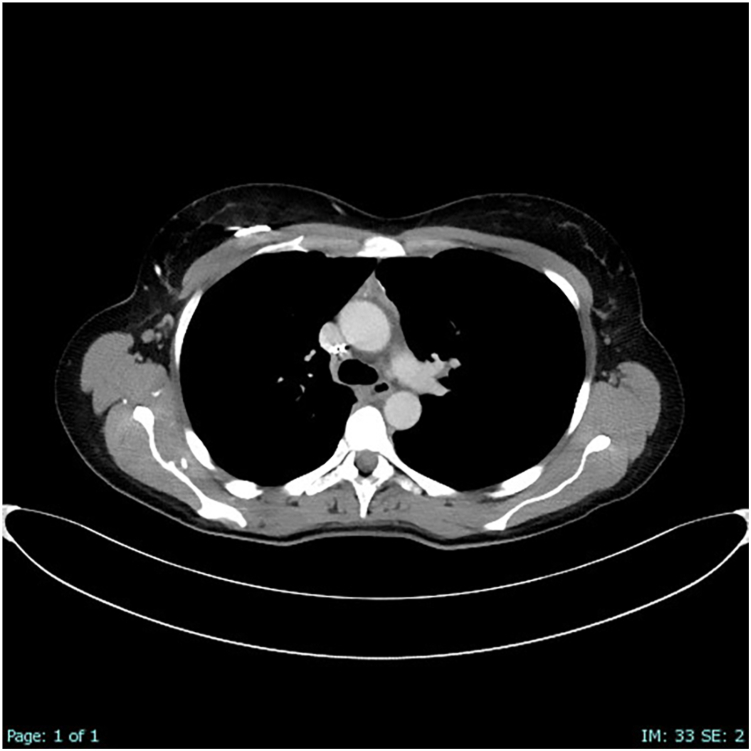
Computed tomography scan of the thorax 6 months after treatment.
